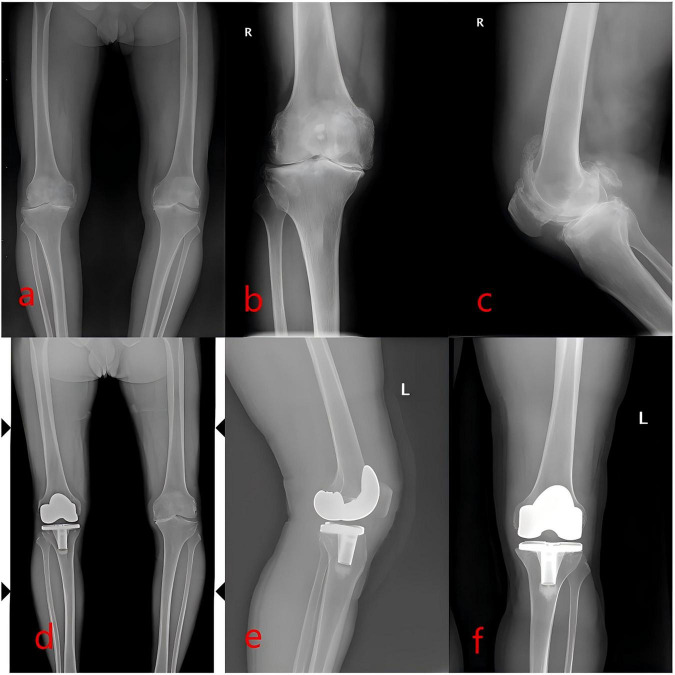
Total knee arthroplasty (TKA). Male patient, 62 years old. (a–c) Preoperative anteroposterior and lateral views of the right knee joint and full-length X-ray of the lower extremity showed the KOA manifestations of the right knee joint, with severe narrowing of the medial compartment of the knee joint and genu varum. (d–f) Postoperative anteroposterior and lateral views of the right knee joint and full-length X-ray showed that the prosthesis was well fixed without any loosening signs. The genu varum and narrowing of the knee joint were corrected, and the lower extremity alignment was improved compared to that before the operation.
